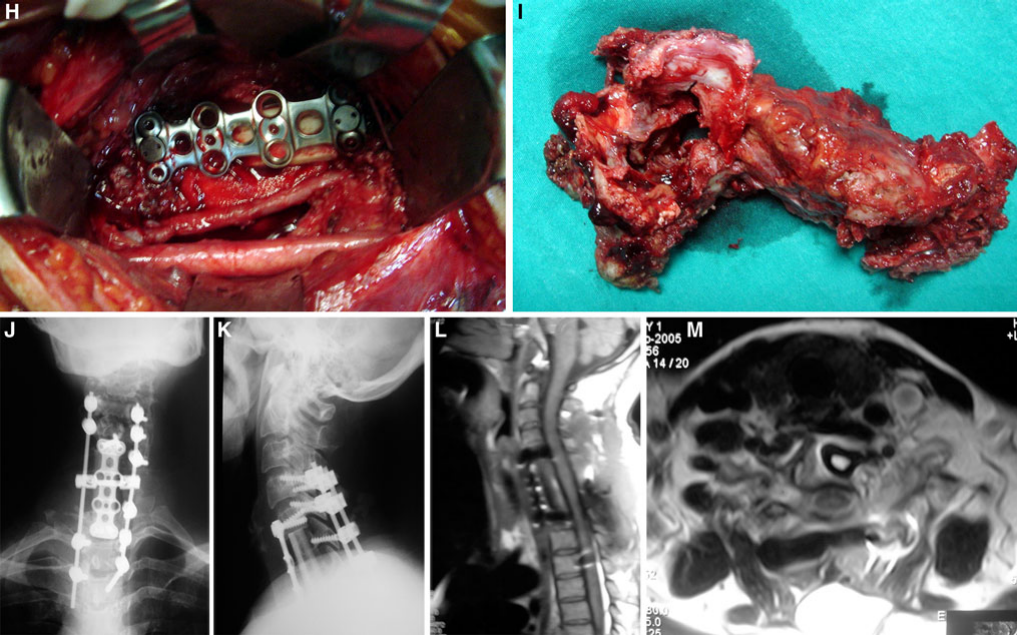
a–m Case # 2 A 46-year-old female came with neck pain, left arm weakness for 4 months. Neurological examination showed a monoparesis of left upper extremity. MR and CT scans showed a tumor on the left side of C6 and C7 vertebral bodies. C6, C7 body, C7 lamina, pedicle, facet joint, left C6–C7 neural foramina. WBB scale was 1–8, 12 ABCD. A total spondylectomy was performed using a combined posterior, anterior and posterior approach. Spinal reconstruction was achieved using a fibula allograft, anterior cervical plate and posterior lateral mass screw-rod system. There was no recurrence during the 16-month follow-up period
